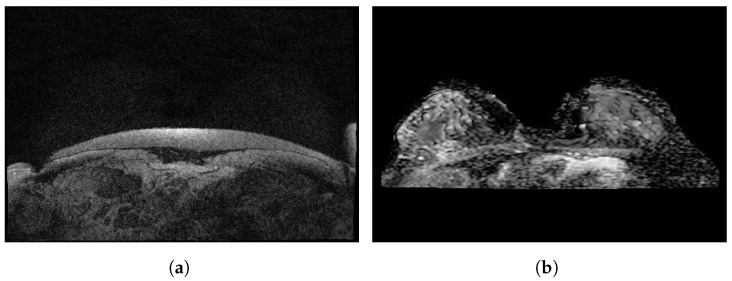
Sample images from (a): Duke-Breast-Cancer-MRI database; (b): RIDER Breast MRI database.
